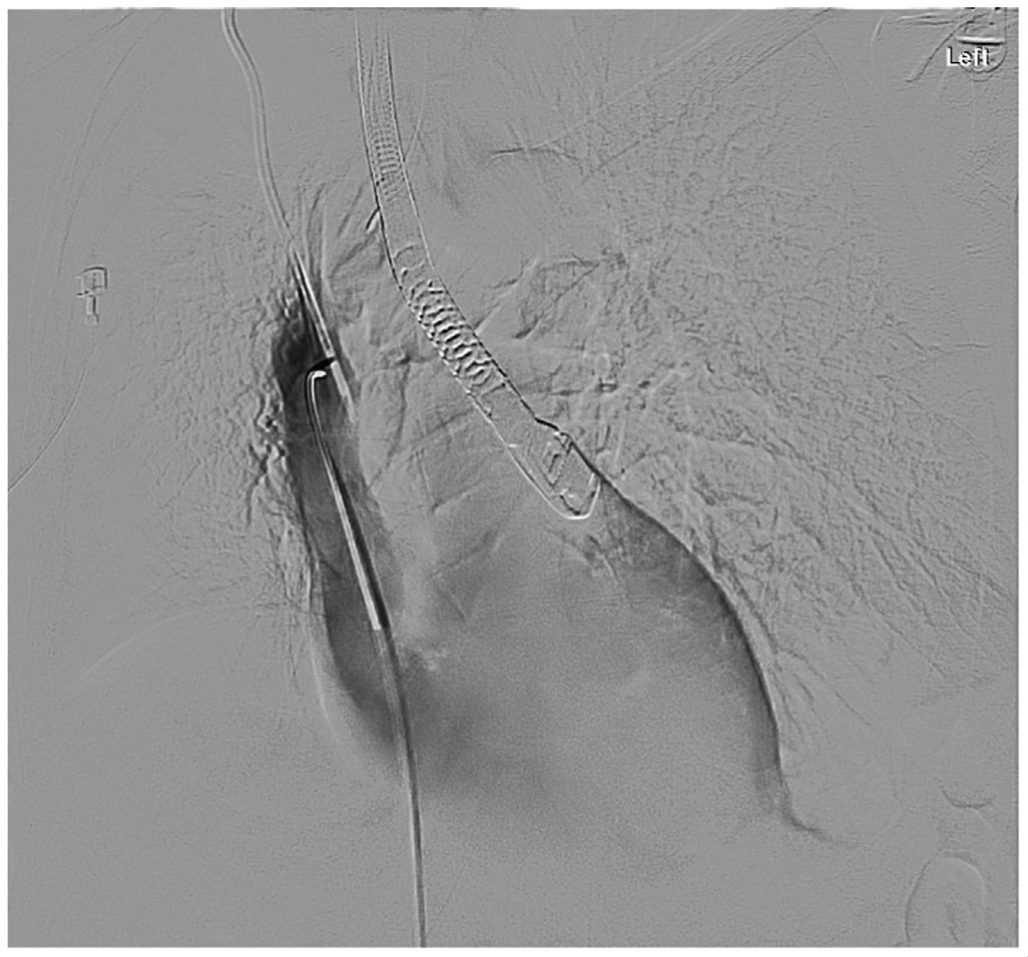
Digital subtraction imaging demonstrating the positioning of the echocardiogram probe and vascular catheters to assist in positioning to locate the clot-in-transit (CIT).
